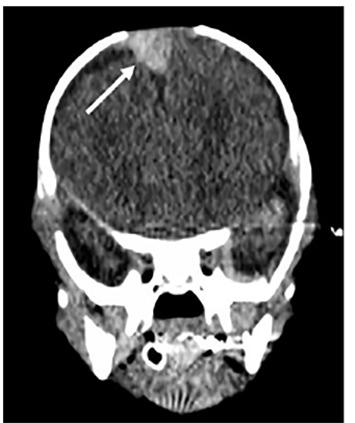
Non-contrast computed tomography demonstrating a 1.2 x 1.3 centimeter subdural hematoma (arrow).
